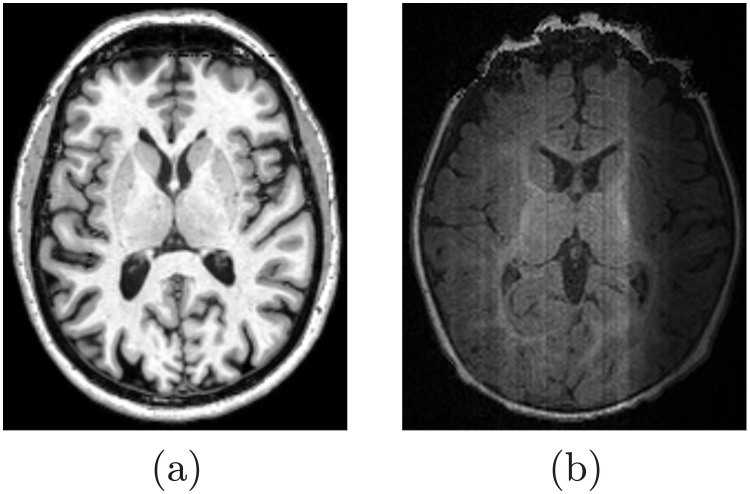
T1-weighted MRI for adult (a) and infant (b) brains.
